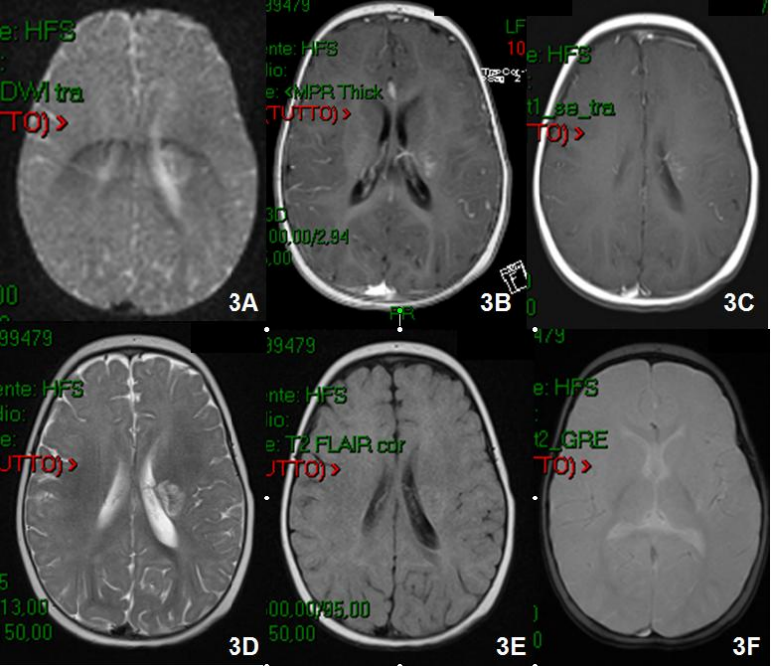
Brain MRI with Gd-DTPA performed 45 days after the first one showed a reduction of the left lenticular nucleus lesion.
